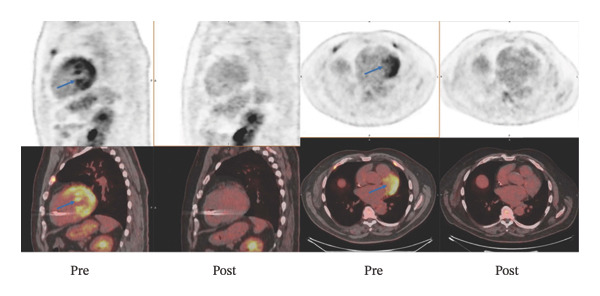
Representative sagittal and axial PET and PET/CT fusion images in a patient with cardiac sarcoidosis. The top row shows baseline PET images with increased FDG uptake in the left ventricular myocardium (blue arrows), indicating active inflammation. The bottom row displays corresponding fused PET/CT images. Posttreatment scans (second and fourth columns) demonstrate a significant reduction in myocardial FDG uptake, consistent with the metabolic response to immunosuppressive therapy.
